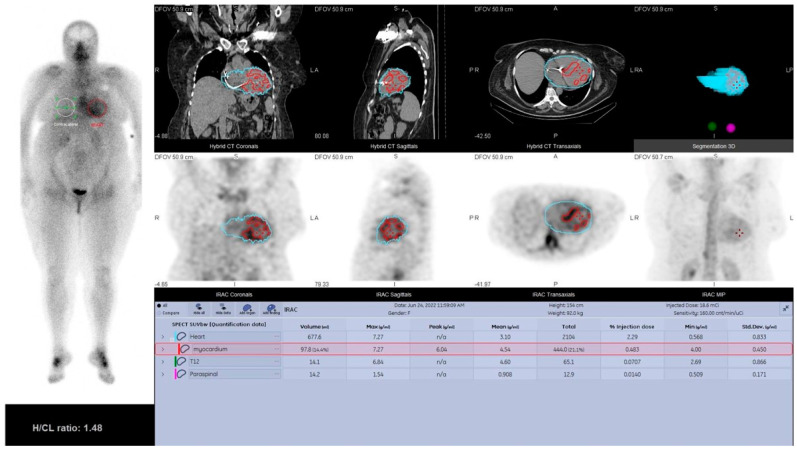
47 year old patient diagnosed with familial ATTR (Glu54Gln). The planar bone scan shows ^99m^Tc-PYP cardiac uptake greater than bone uptake, transcribing into Perugini score 3. Quantitative SPECT/CT confirms this observation, with the myocardium showing an SUV_max_ of 7.27 g/mL, which is higher than the one in bone (namely T12 vertebrae), with SUV_max_ of 6.84 g/mL (Images from our laboratory. Quantitative SPECT/CT was performed using Q.VolumetrixAI software, provided by General Electric Healthcare, on images obtained 3 h post-radiotracer administration. The heart volume was manually segmented and a region-of-interest for measuring the SUV_max_ of the myocardium was inserted and adjusted to the myocardial uptake).
